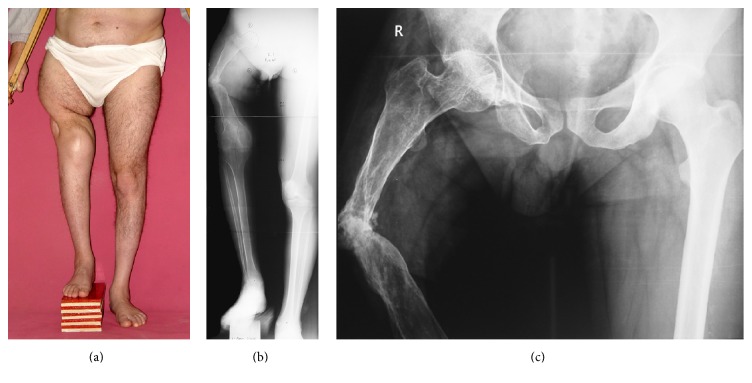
(a) Preoperative clinic appearance with 10 cm length block under the right foot. (b, c) Preoperative radiographs. Note the nonunion and osteoporosis of the right femur.
